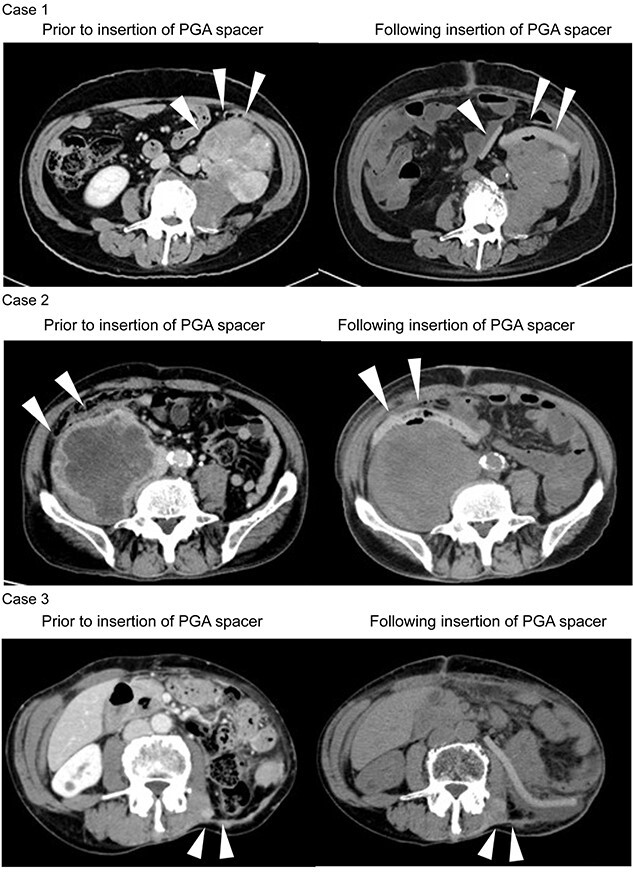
CT images before and after insertion of PGA spacer. Arrows: Location where there is contact between the tumor and the digestive tract.
